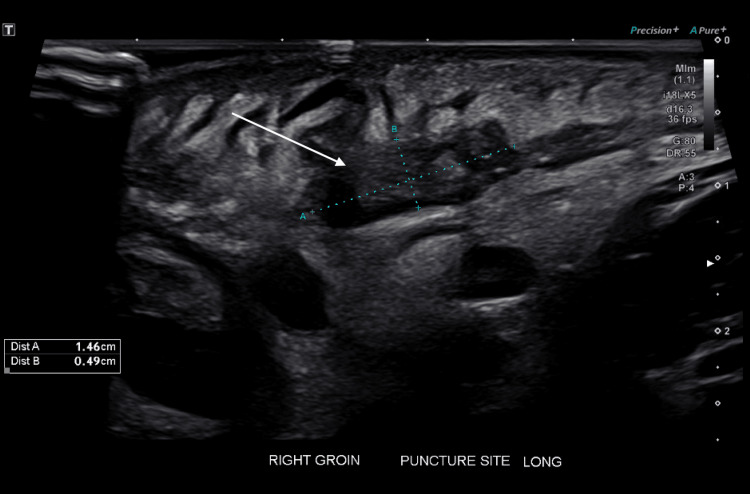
Longitudinal ultrasound of the right thigh (day 8) Linear heterogeneous lobular subcutaneous collection measuring 2.2 × 1.5 × 0.5 cm concerning for abscess (arrow), without a clearly visualized foreign body. The image was acquired using a high‑frequency linear transducer (e.g., 10-15 MHz) at a depth of approximately 3-4 cm with focal zones placed over the area of maximal tenderness.
